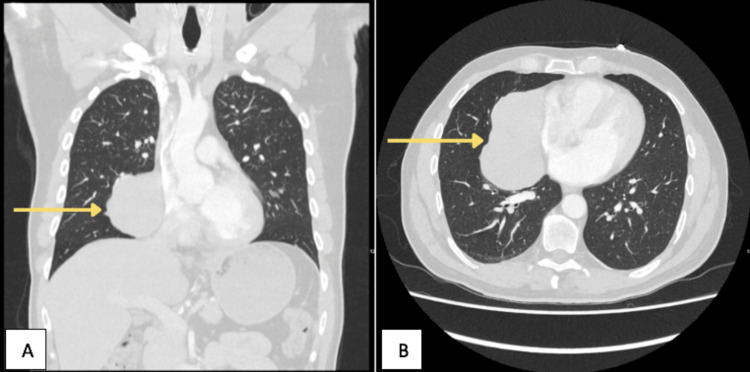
CT chest, lung window; coronal view (A) and axial view (B) showing a solid right-sided thoracic mass with a wide base abutting the pericardium (yellow arrow) and normal background lung parenchyma
